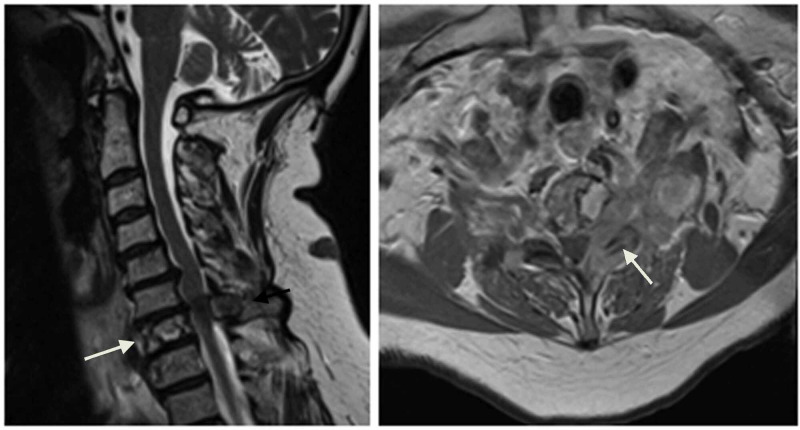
Left: Sagittal T2-weighted image of the cervical spine showing soft tissue mass invading C7 vertebral body and spinous process (arrow). Right: Axial post-contrast T1 weighted image of the cervical spine showing tumor extension into C67 neural foramen (arrow).
